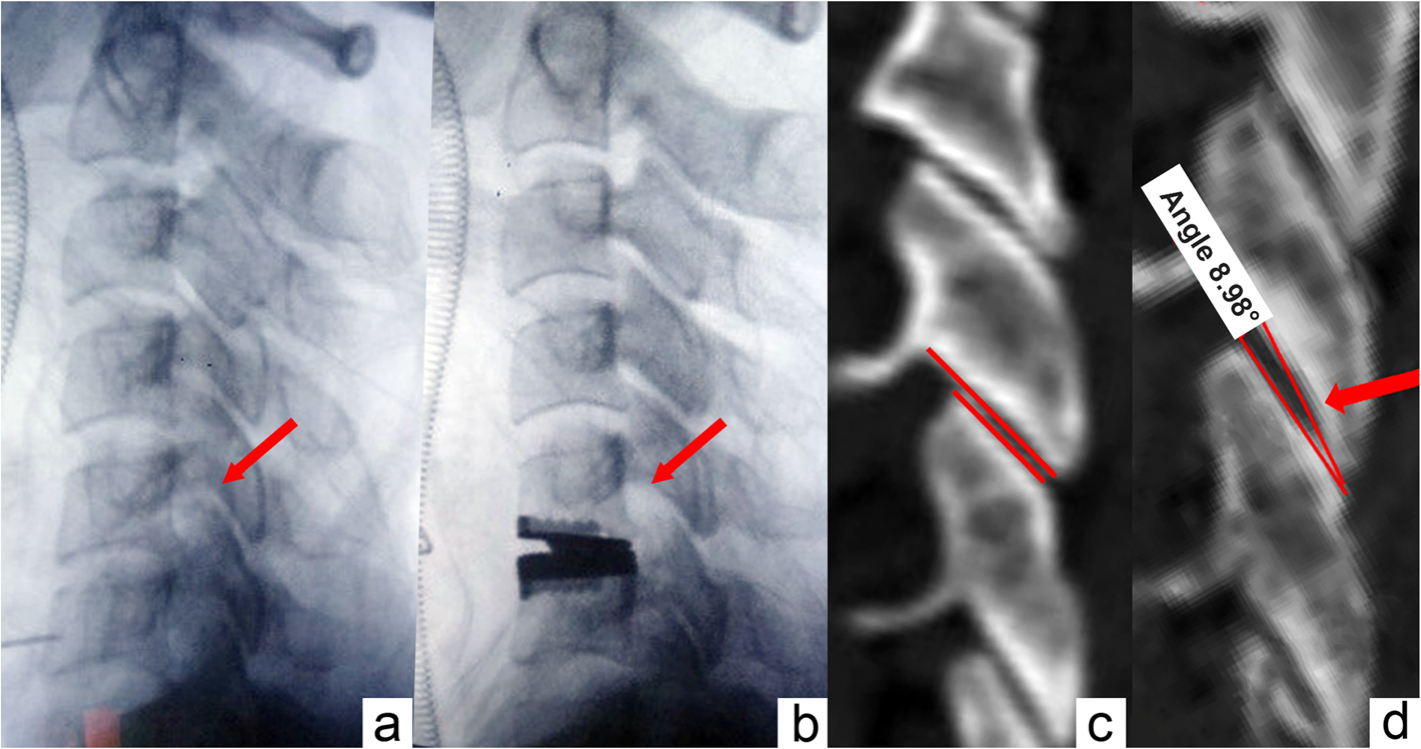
In clinical practice, we may encounter the situation that the facet joint gaps at the index level were stretched by an oversized artificial disc a, b the facet joints are labeled with arrows). Besides, the normal articular surfaces were parallel which could be observed in CT scan c, and we found that with the increment of intervertebral height, the articular surfaces at index level would form a certain angle d the angled facet joint is labeled with an arrow)
